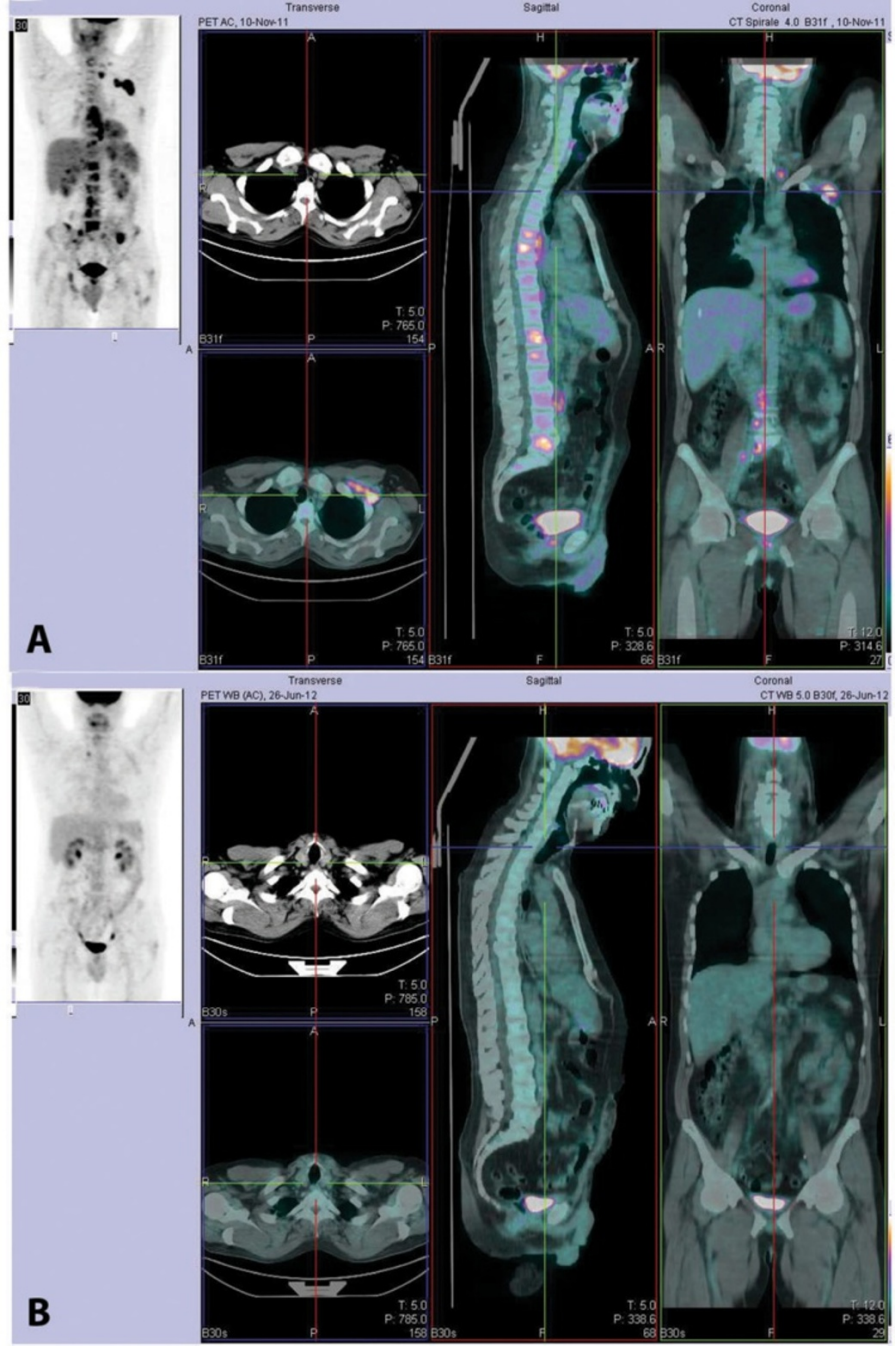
FDG PET scan images before and after treatment. (A) Staging FDG PET scan image showing nodal disease on both sides of the diaphragm with enhanced metabolic activity in the spleen and skeleton. (B) End of treatment: FDG PET image showing no residual disease.
